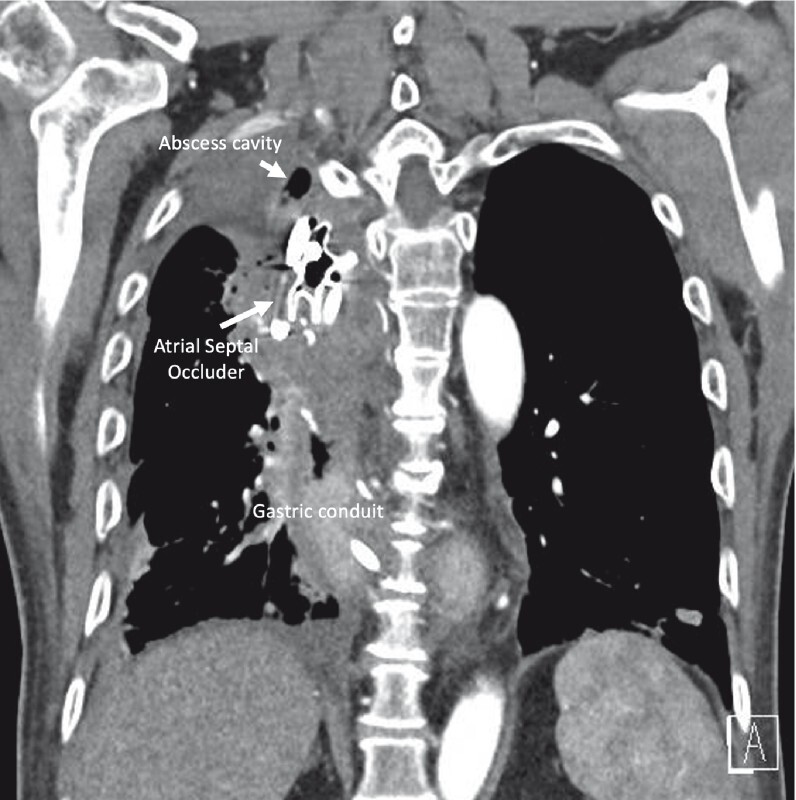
Interval computed tomography scan at 1 week showing the atrial septal occluder device positioned between the abscess cavity and neo-esophagus.
